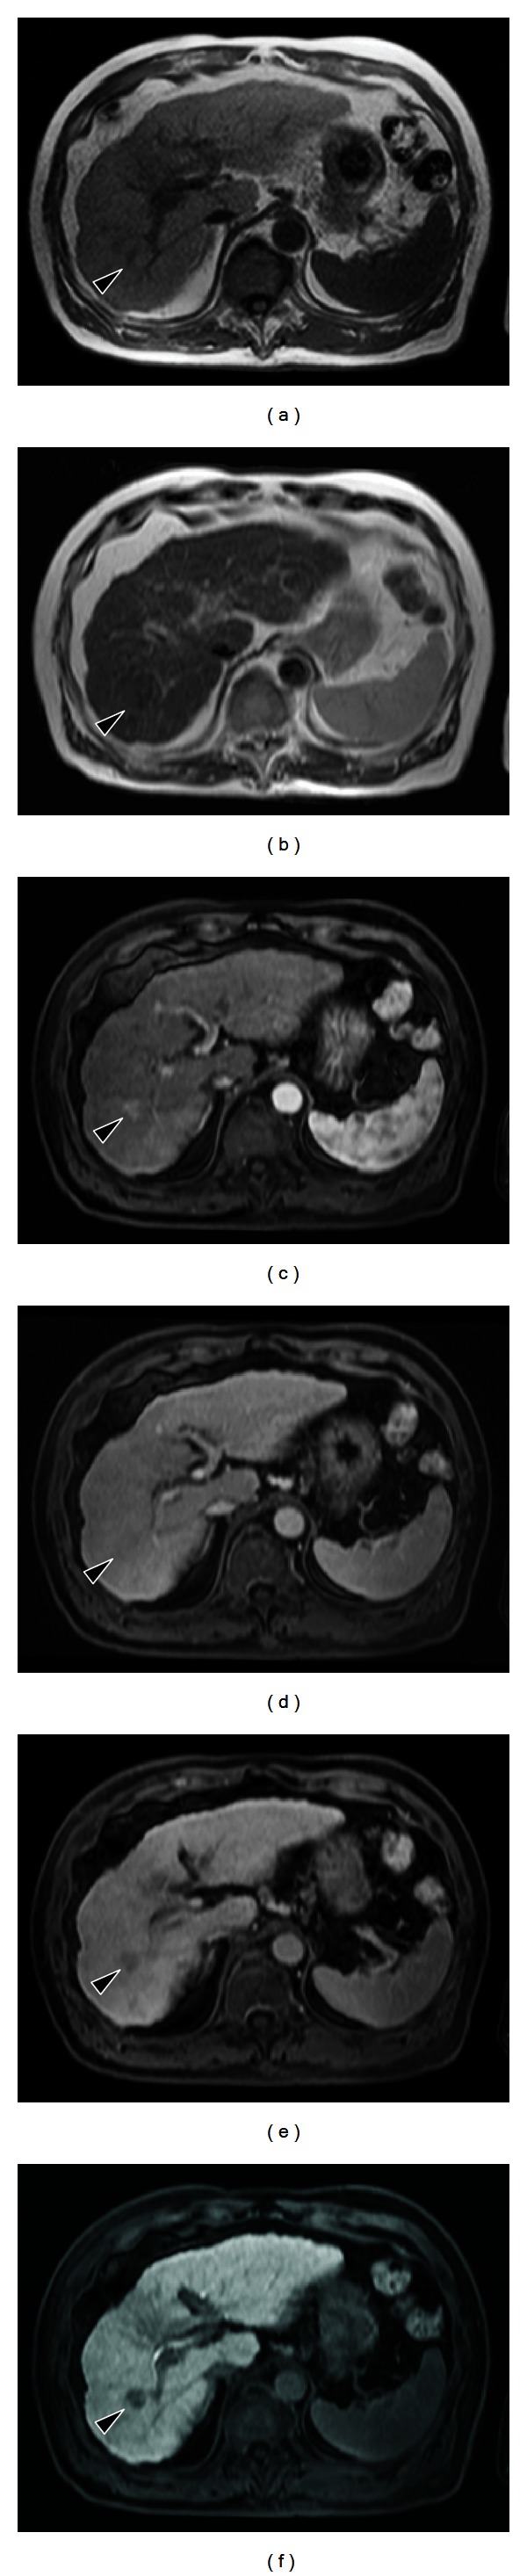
MR images of hepatic inflammatory pseudotumor. (a) The lesion appears isodense (arrow) on T1-weighted image. (b) A partially round, hyperintense lesion (arrow) is observed on T2-weighted image. ((c)–(f)) Gadolinium ethoxybenzyl diethylenetriamine pentaacetic acid-enhanced images. In the arterial phase following contrast injection (c), the center of the tumor is enhanced intensely and homogeneously. In the delayed phase (d) and hepatobiliary phase (f), the tumor is completely washed out. (e) portal phase.
